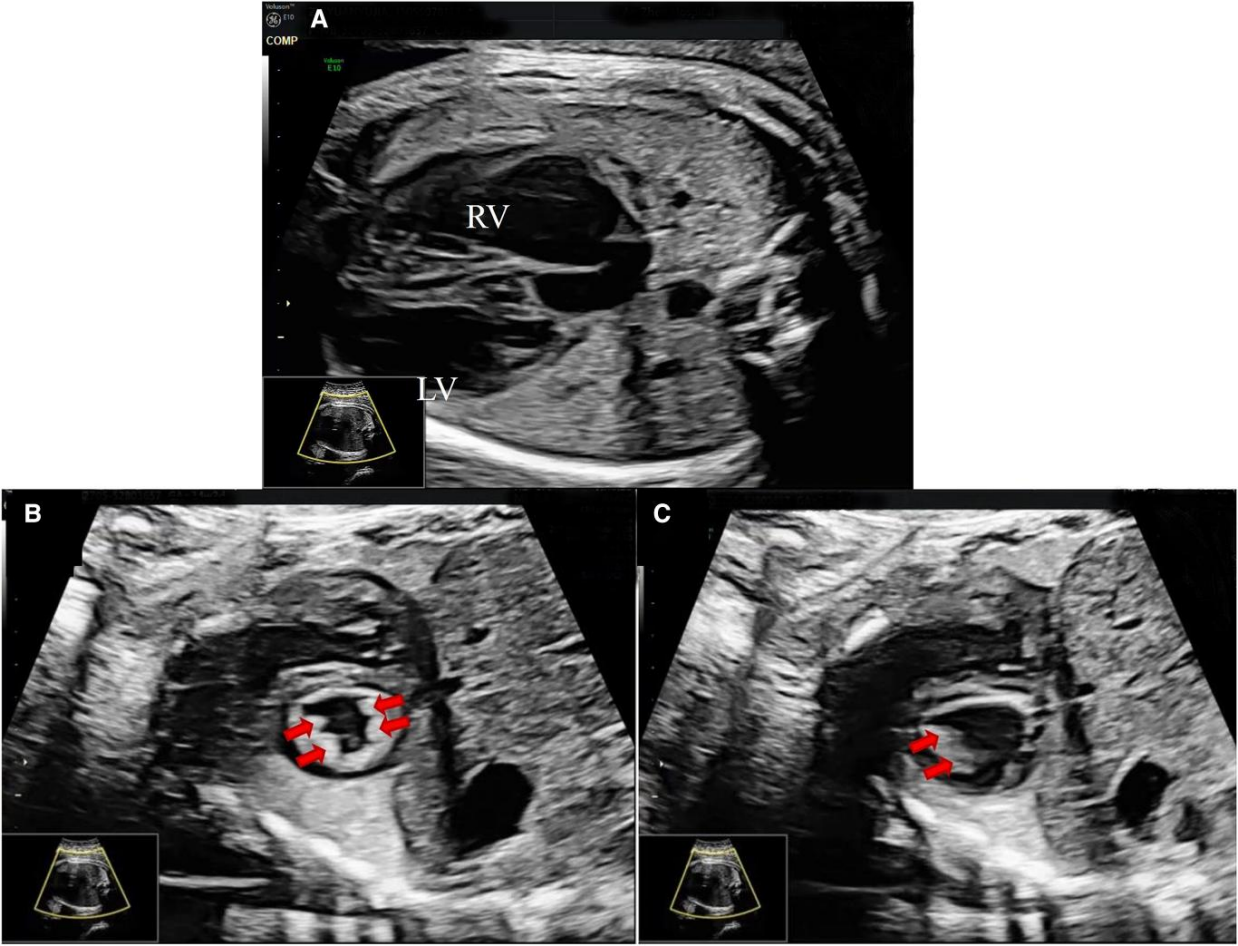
(A)* Left ventricular enlargement. *(B)* Foetal echocardiography of the short axis in the systolic phase. Saw-tooth appearance of myocardium (arrows) in basal inferolateral and basal to mid lateral segment *(C)* Diastolic phase in a short axis section shows saw-tooth appearance of myocardium (arrows) in basal inferolateral.
